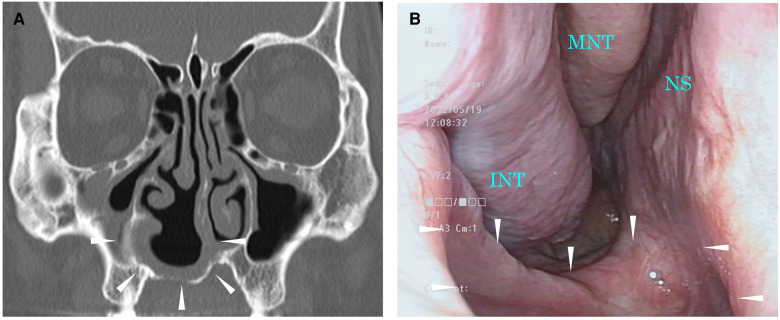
Images 3 months after surgery. The area surrounded by the arrowhead indicates a surgically opened area. (A) CT scan of the sinuses. (B) Endoscopic image of the right nasal cavity. CT, computed tomography; INT, inferior nasal turbinate; MNT, middle nasal turbinate; NS, nasal septum.
